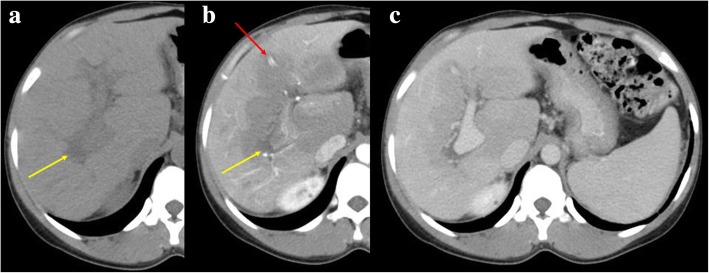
Obliterative portal venopathy. 32-year-old female, with HIV infection and slightly increased bilirubin levels, who complained of increased abdominal volume. Axial (a) plain, (b) arterial, and (c) portal venous phase contrast-enhanced CT images show some morphologic liver changes, with hypertrophy of the caudate lobe, but regular contours and no atrophy of segment IV. Right PV is hypoattenuating before and after contrast material administration (yellow arrows), a feature suggesting non-acute thrombus. Hepatic parenchyma displays heterogeneous peripheral enhancement in arterial phase (b) due to compensatory increased arterial flow. This THED fades in portal venous phase (c). Some PH stigmata are present, as such splenomegaly, permeable umbilical vein (red arrow), and other varices. Liver biopsy was performed and OPV diagnosis pathologically confirmed
